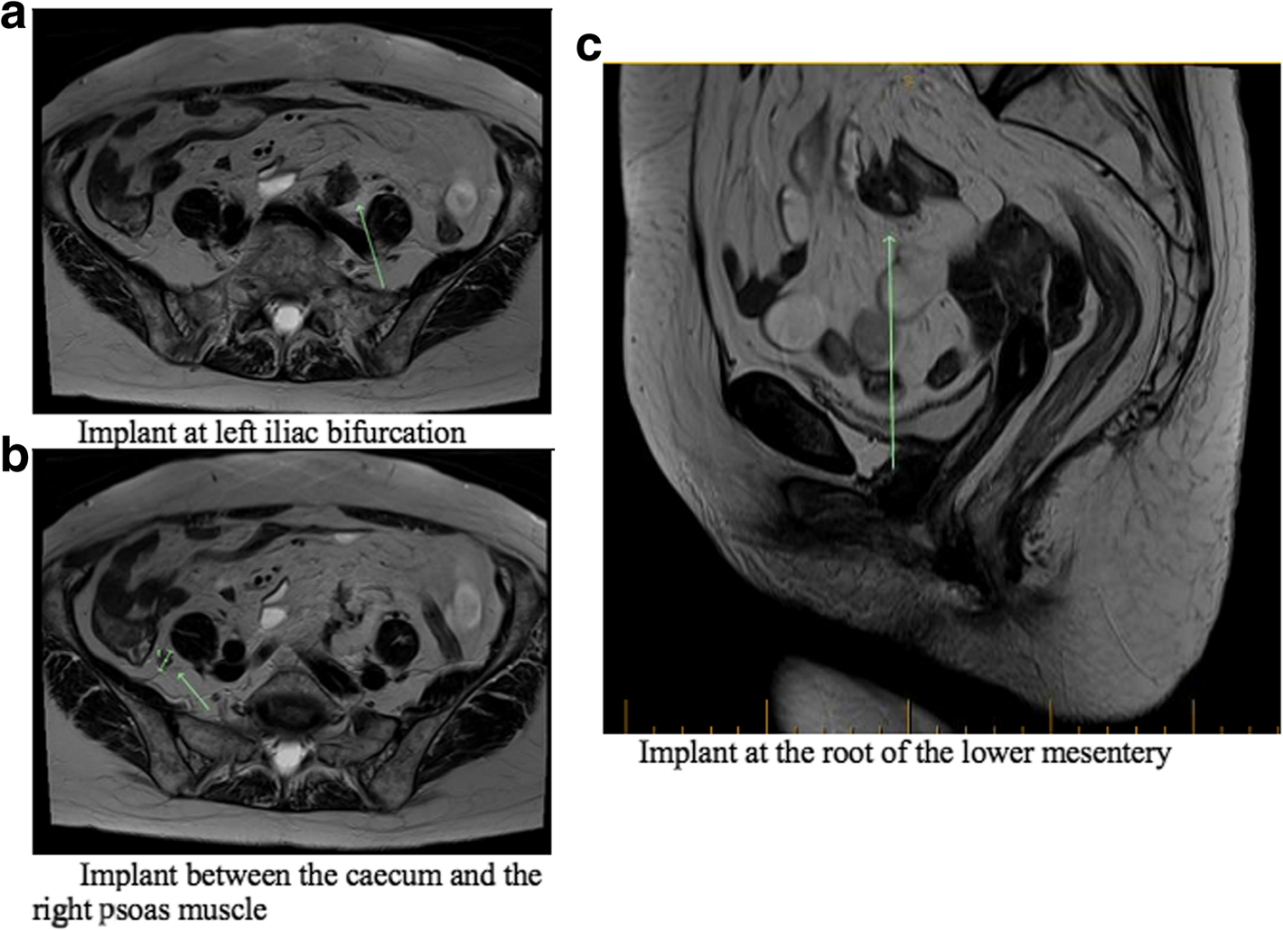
MRI axial and sagittal images showcasing the peritoneal carcinomatosis implants in the abdomino-pelvic region
